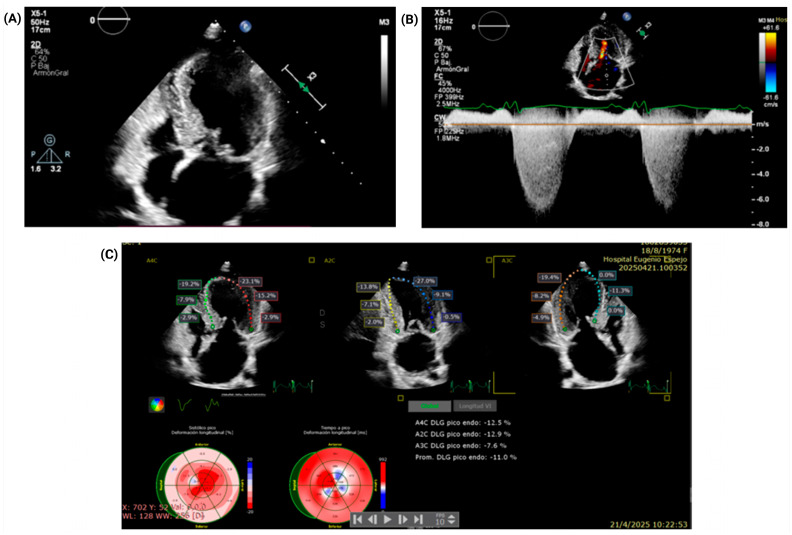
Transthoracic echocardiography: structural, hemodynamic, and deformation findings. (A) Two-dimensional transthoracic echocardiography demonstrating asymmetric septal hypertrophy, predominantly involving the anteroseptal and inferoseptal walls (basal to mid-ventricular segments), with a maximal end-diastolic wall thickness of 18 mm. (B) Doppler assessment of mitral inflow showing estimated peak and mean diastolic left atrial–left ventricular gradients of 19 mmHg and 6 mmHg, respectively; severe mitral regurgitation was present, with a peak systolic left ventricular–left atrial regurgitant velocity of 6.4 m/s, consistent with systolic anterior motion-related mitral regurgitation. (C) Speckle-tracking echocardiography demonstrating markedly reduced global longitudinal strain (GLS), with a reported GLS value of −11%.
